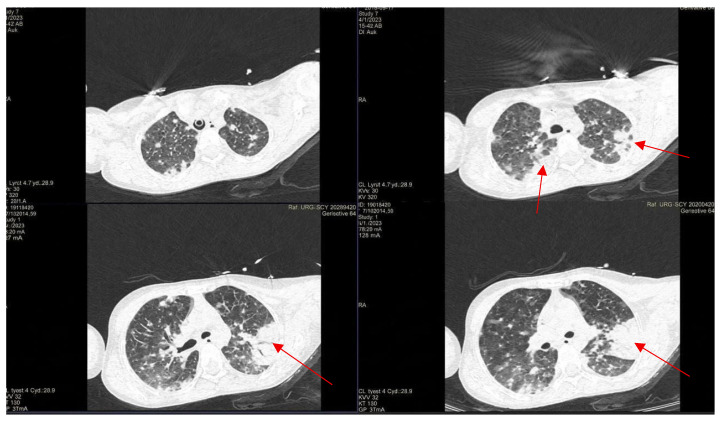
Non-contrast chest computed tomography (CT), axial view, lung window. The images demonstrate consolidated lesions in the right upper, left upper, and lower lobes. Additionally, multiple diffusely distributed foci of nodular consolidation are present throughout both pulmonary hemifields. Red arrows highlight the prominent areas of consolidation.
